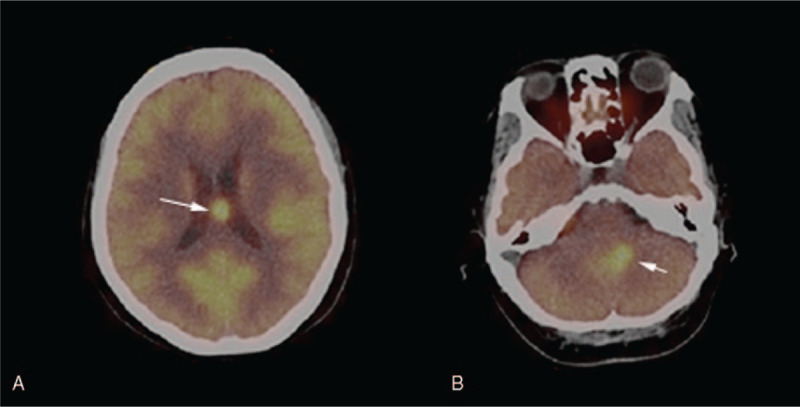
FDG-PET performed 4 months before this presentation showed that FDG uptake increased in the callosal area with a maximal SUV of 16.5 (panel A), and the area abutting the fourth ventricle with a maximal SUV of 16.2 (panel B).
